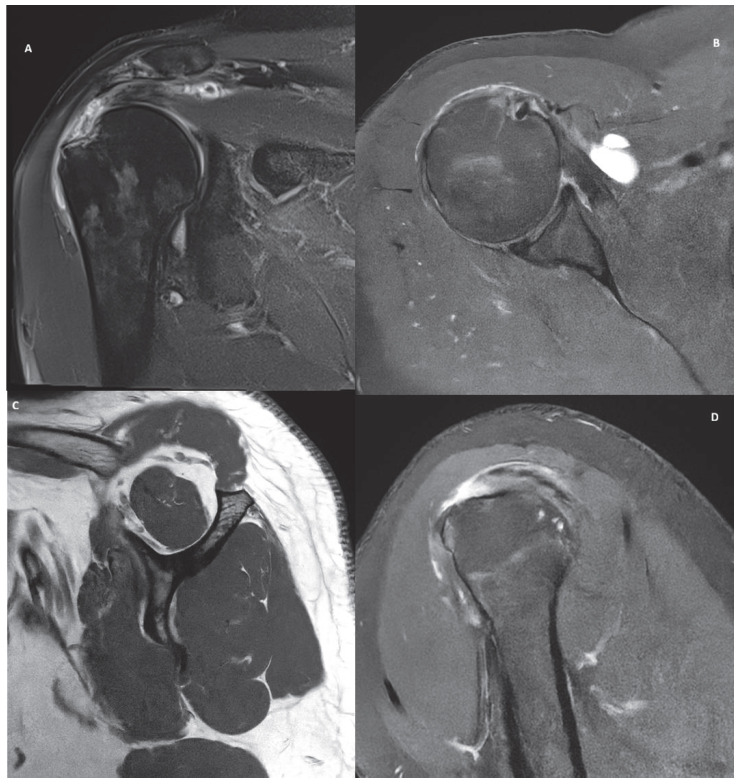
MRI images of an isolated supraspinatus full thickness tear in coronal (A), axial (B) and sagittal (C,D) view.
